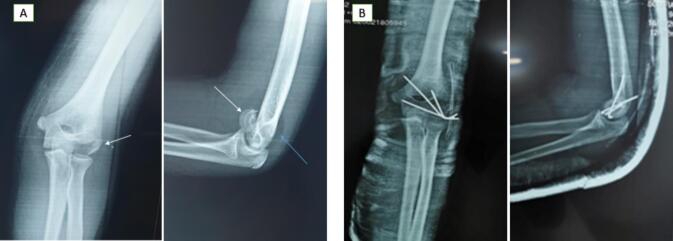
Case 3: Anterior Capitellar Shear Fracture (Type IV - McKee) with Proximal and Lateral Displacement. (A) Anteroposterior radiograph showing a lateral fracture fragment (black arrow). Lateral X-ray revealed a fat pad sign (blue arrow) and a double contour sign. (B) Postoperative anteroposterior and lateral radiographs demonstrating proper reduction and K-wire placement. (For interpretation of the references to colour in this figure legend, the reader is referred to the web version of this article.)
